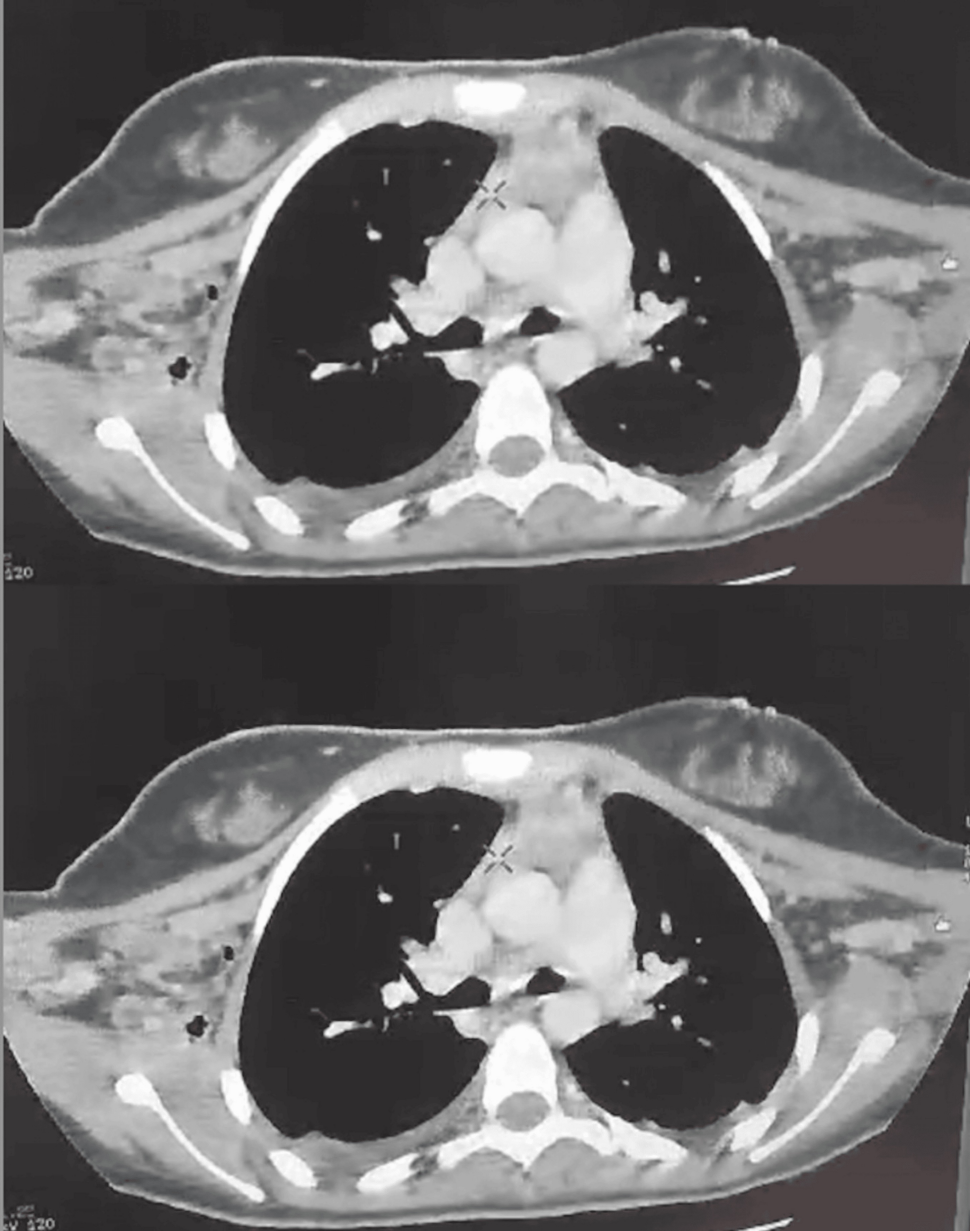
Postoperative CT Scan of Our Patient With Descending Necrotizing Mediastinitis (Day 3) This postoperative CT scan, performed on day 3 after surgery, showed the absence of new fluid collections or significant mediastinal widening, suggesting a favorable postoperative evolution
